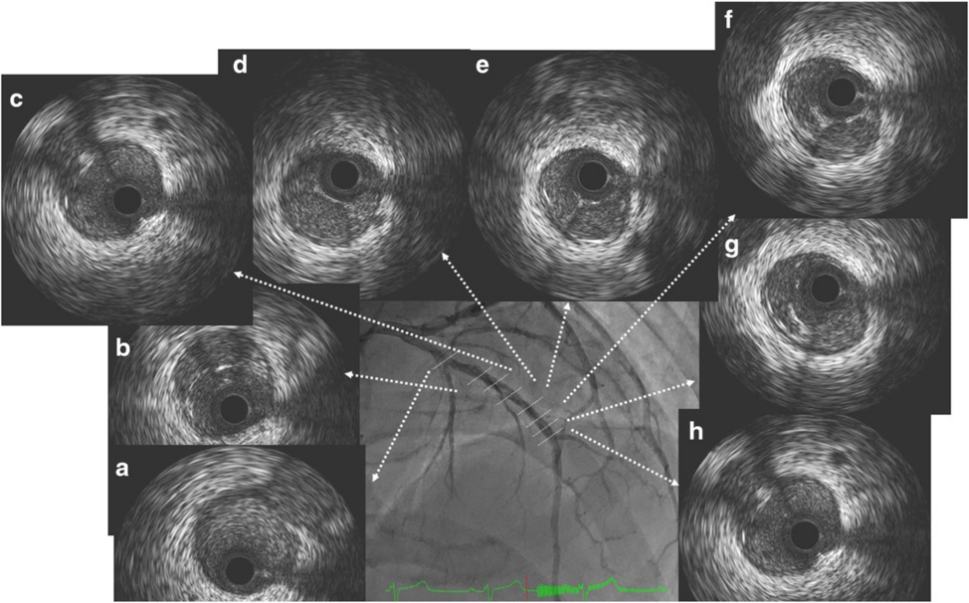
Intravascular ultrasound image demonstrating the honeycomb-like structure in the mid left anterior descending coronary artery (b–h). No atheromatous plaques were seen (a)
